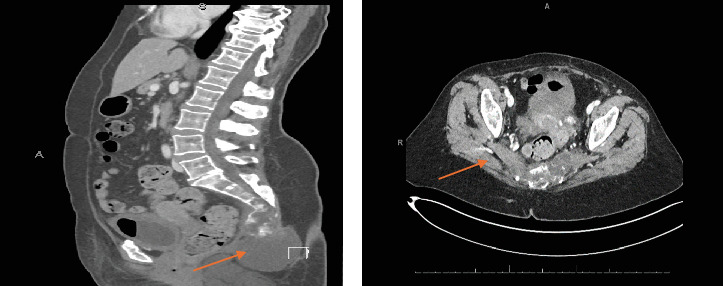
Preoperative CT scan showing an ill-defined soft tissue mass (arrows) in the coccygeal and lower sacral region. (a) Sagittal view. (b) Coronal view.
